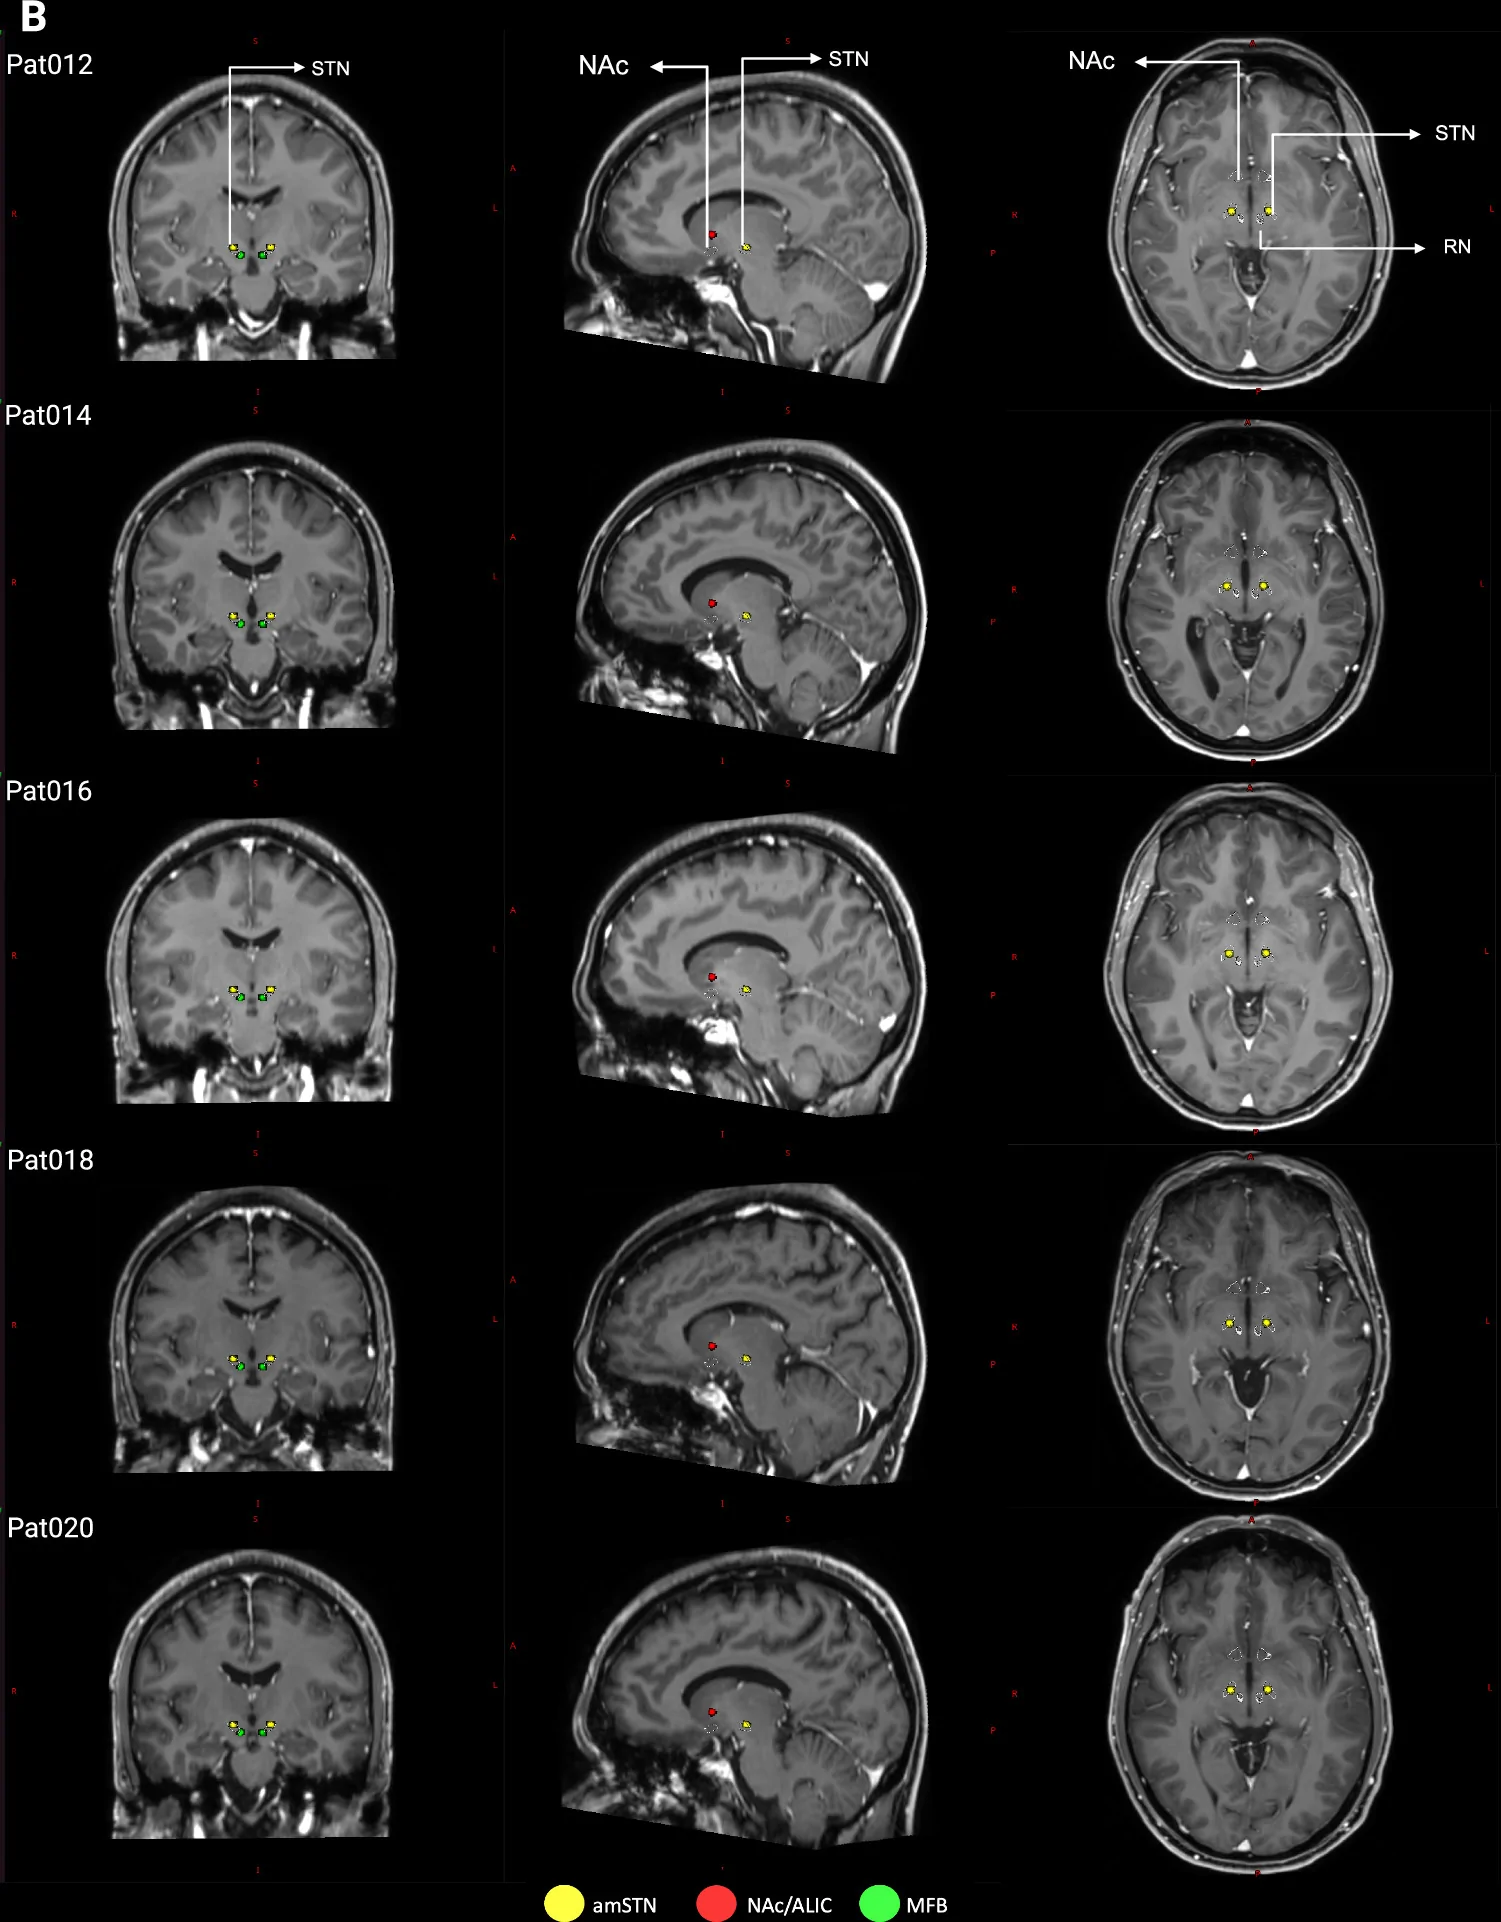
A and 1B. Patient-specific localisation of standardised spherical ROIs representing trOCD-DBS targets in native anatomical space. Native space localisation of three trOCD-relevant DBS targets, the nucleus accumbens/anterior limb of the internal capsule (NAc/ALIC, red), the anteromedial subthalamic nucleus (amSTN, yellow), and the medial forebrain bundle (MFB, green), in ten randomised participants. Figure 1A shows the odd-numbered participants (Pat01, Pat03, Pat05, Pat07, Pat09) and 1B the even-numbered participants (Pat012, Pat014, Pat016, Pat018, Pat020); each row displays coronal, sagittal, and axial views of the native T1-weighted image. Bilateral 2 mm radius spherical ROIs were centered on each participant’s patient-specific target coordinates, and anatomical annotations in the first row of each panel indicate the nucleus accumbens (NAc), subthalamic nucleus (STN), and red nucleus (RN). Identically sized ROIs standardised the spatial sampling volume across targets and participants, enabling direct comparison of the subsequent structural-connectivity analyses.
